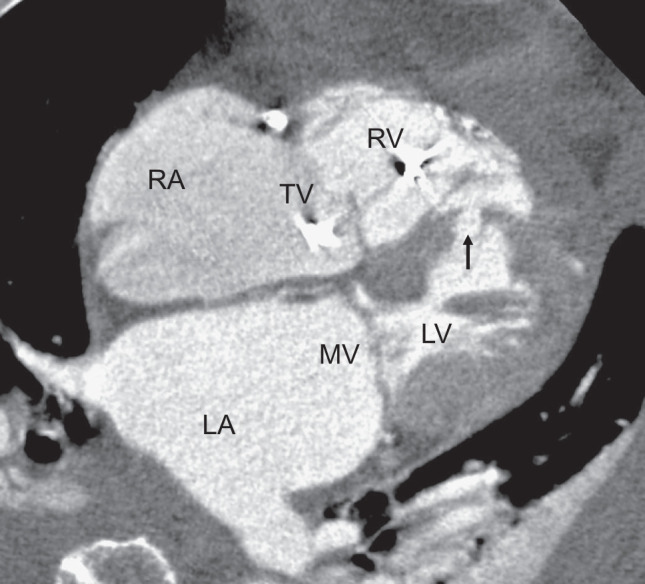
Cardiac CT. A 4-chamber cardiac CT image showed a myocardial defect at the posterior wall of the interventricular septum (arrow). The VSR was located near the apex and was kept away from the subvalvular apparatus of the mitral and tricuspid valves. LA, left atrium; LV, left ventricle; MV, mitral valve; RA, right atrium; RV, right ventricle; TV, tricuspid valve; VSR, ventricular septum rupture
